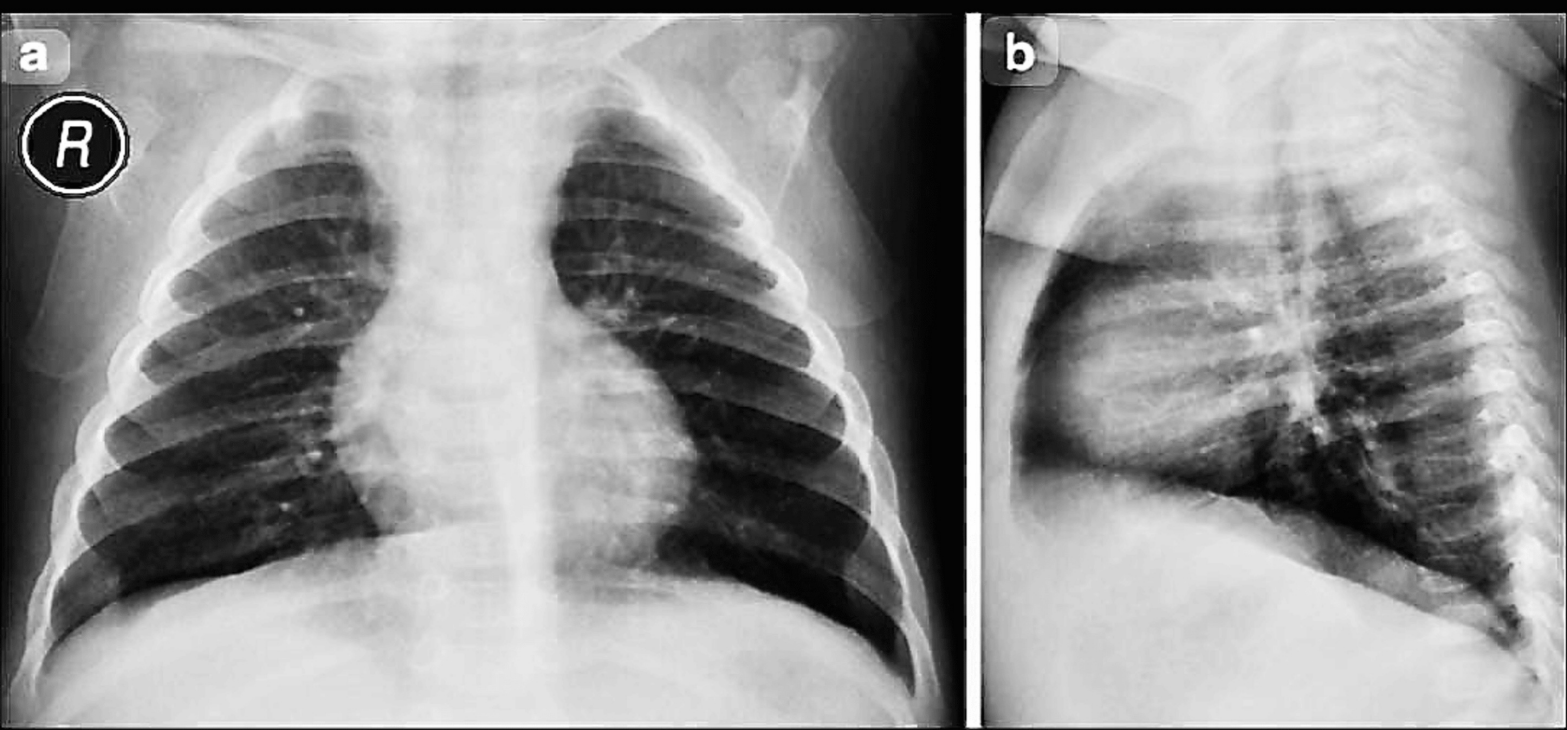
Posteroanterior and lateral chest radiographs of the pediatric patient Chest radiograph of an 18-month-old female presenting with pneumonia. (a) Posteroanterior view showing bilateral pulmonary opacities, predominantly involving the right middle and lower zones, consistent with alveolar consolidation. The cardiac silhouette appears normal for age, and no pleural effusion or pneumothorax is seen. (b) Lateral view further confirms consolidation in the posterior segments of the right lower lobe, supporting the diagnosis of lobar pneumonia.
